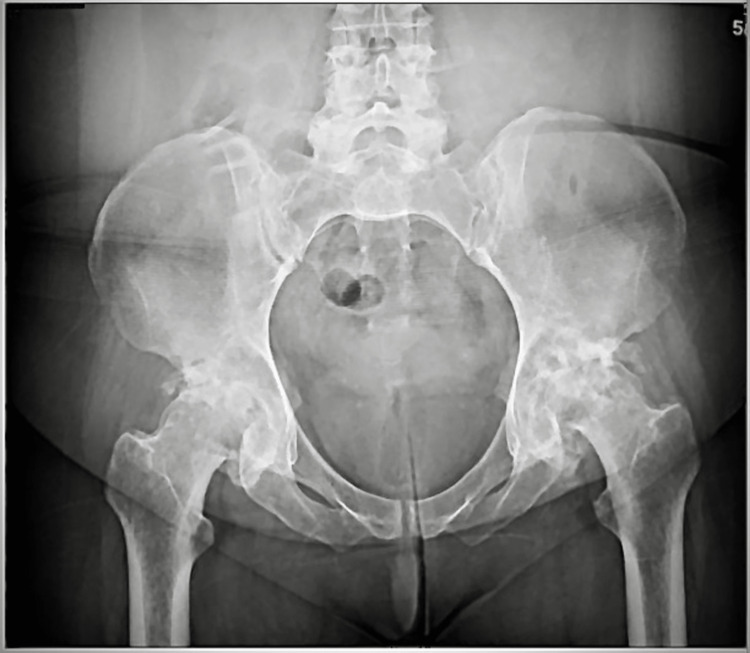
Bilateral Hip X-ray Plain radiograph suggesting osteonecrosis of the femoral head with evident sclerosis and joint space narrowing bilaterally.
